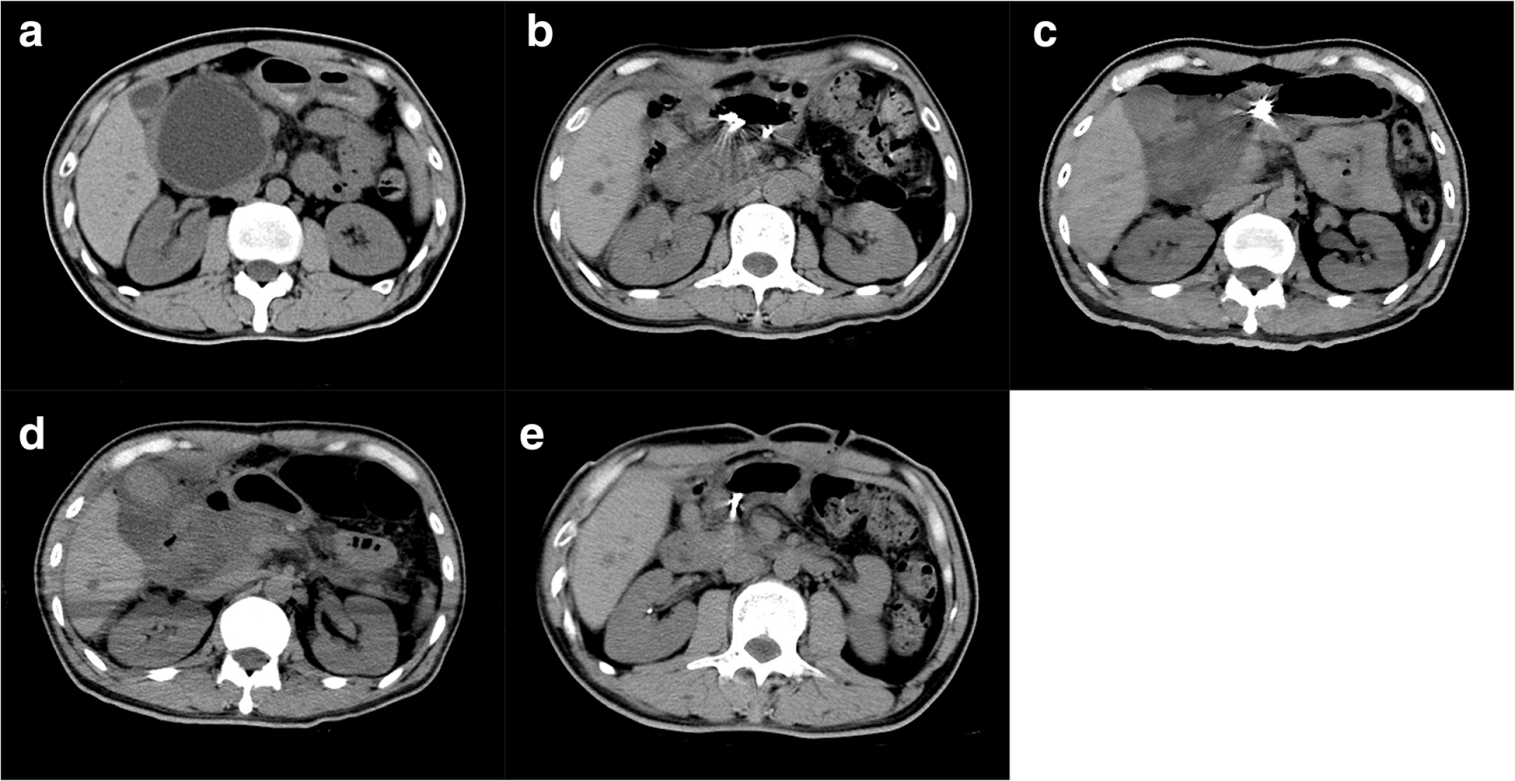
A PPC case who first received endoscopic cystgastrostomy complicated with anastomotic leakage. The patient received surgical drainage 4 days after endoscopy. a Abdominal CT scan showing a 8-cm PPC; b Pneumoperitoneum occurred on day 1 post-endoscopy; c Seroperitoneum occurred on day 2 post-endoscopy; d Peritonitis occurred on day 3 post-endoscopy; e Pneumoperitoneum and seroperitoneum disappeared in 1 month since surgical drainage
